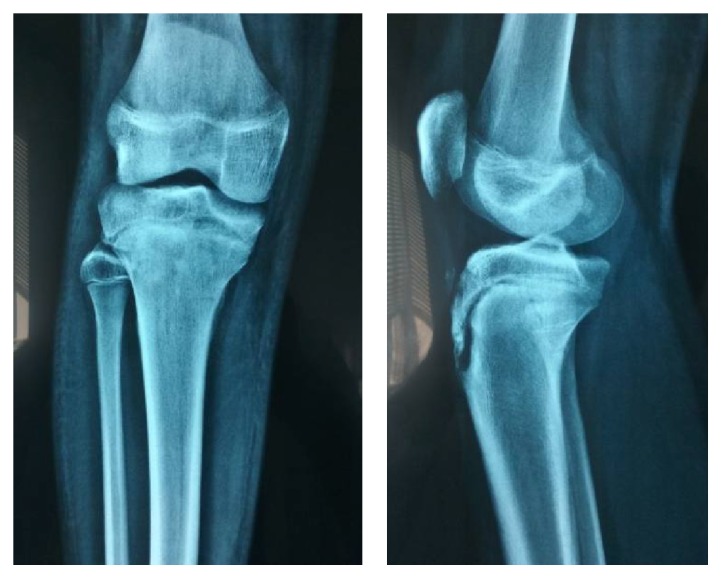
Plain radiographs 6 weeks after reduction.
